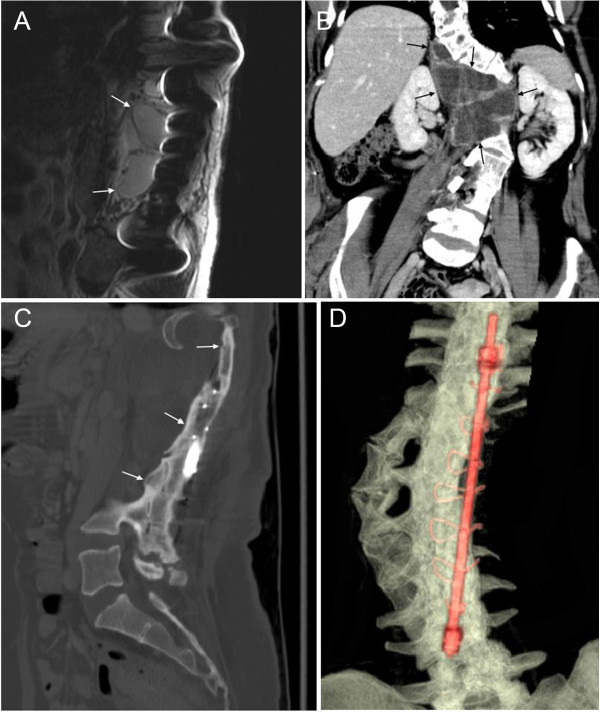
A 20 year-old women (Patient #18) with large neurofibromas and vertebral body dysplasia. A) Sagittal T1 postcontrast MRI demonstrates neurofibromas (arrows). B) Axial T1 postcontrast MR shows the neurofibromas (large arrows) and suggests that a rotatory scoliosis exists. C, D) 3D volume rendered images shows the spinal dysplasia (arrows) and accompanying scoliosis. These images can be rotated and viewed form 360 degrees.
